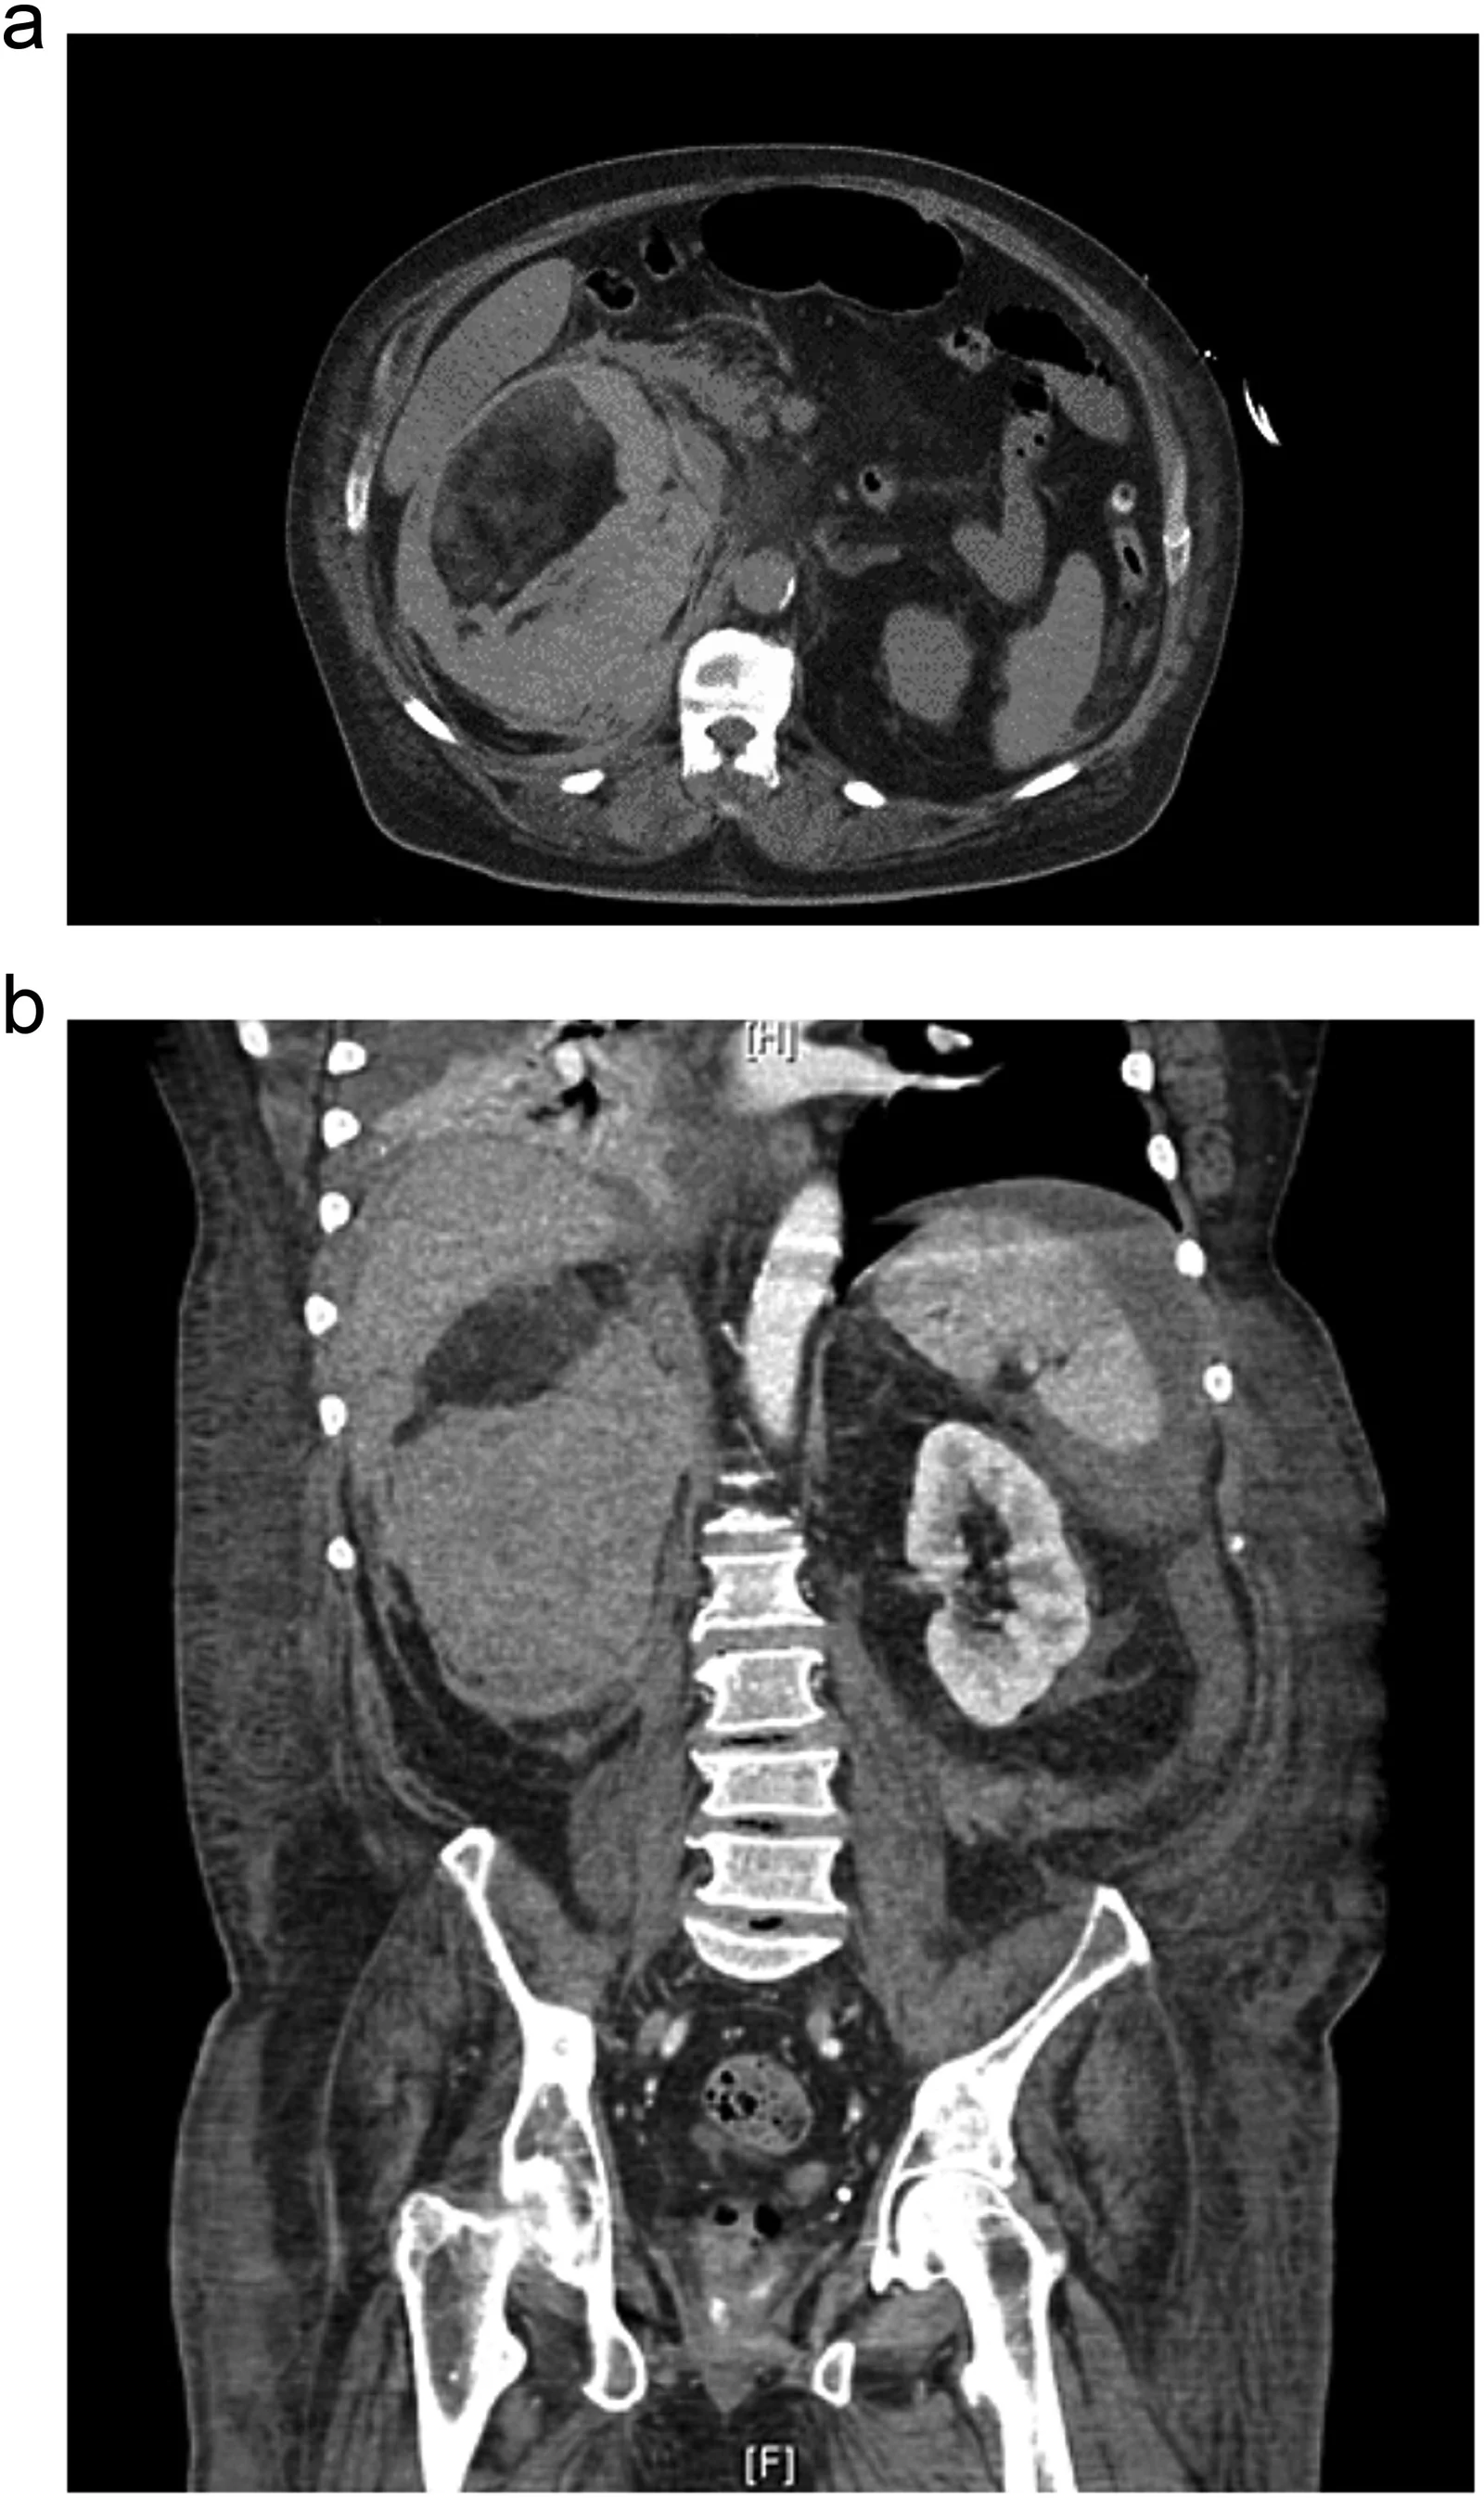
Axial (a) and coronal (b) contrast-enhanced CT images demonstrating a large heterogeneous right adrenal mass with mixed fat and soft tissue components and surrounding hyperdense areas consistent with acute hemorrhage.
